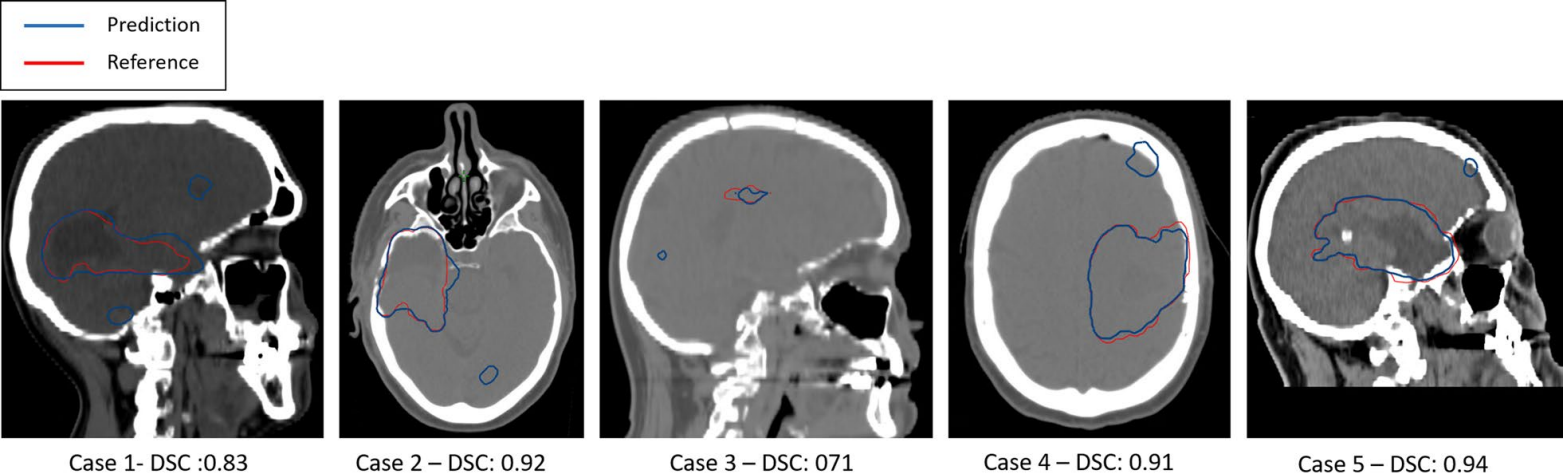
Contours of the predicted PTV (blue) and the reference PTV (red) of the five cases overlaid on an axial or sagittal slice of the planning CT to show the relation between the main target and the outlier. Additionally the dice similarity coefficient (DSC) of the predicted PTV versus the reference PTV is given
